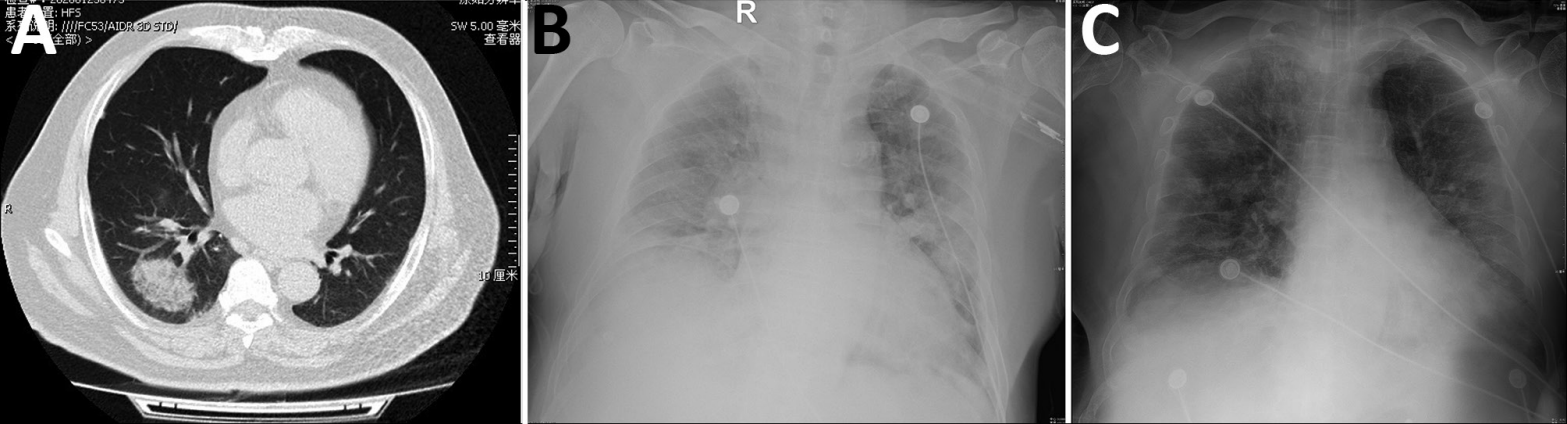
Radiographs of patient co-infected with severe acute respiratory syndrome coronavirus 2 and influenza A virus, China, 2020. A) Chest computed tomography demonstrating a mass, ground-glass consolidation in the right inferior lobe. B) Chest radiograph showing bilateral diffuse exudative shadows, indicating acute respiratory distress syndrome. C) Chest radiograph showing improved lung fields after 4 days in the intensive care unit.
